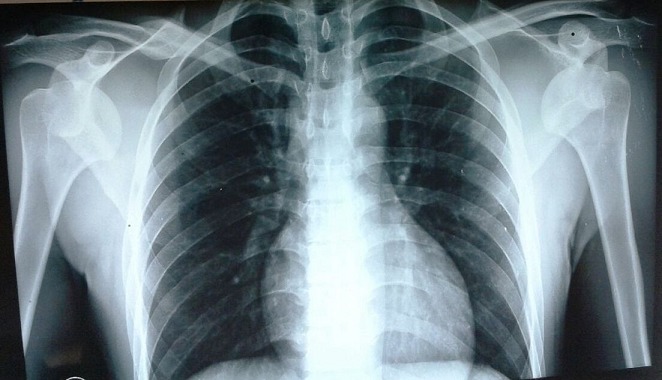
Radiographie des épaules de face montrant la Luxation antéro-interne dans sa variété sous-coracoïdienne chez le premier patient
